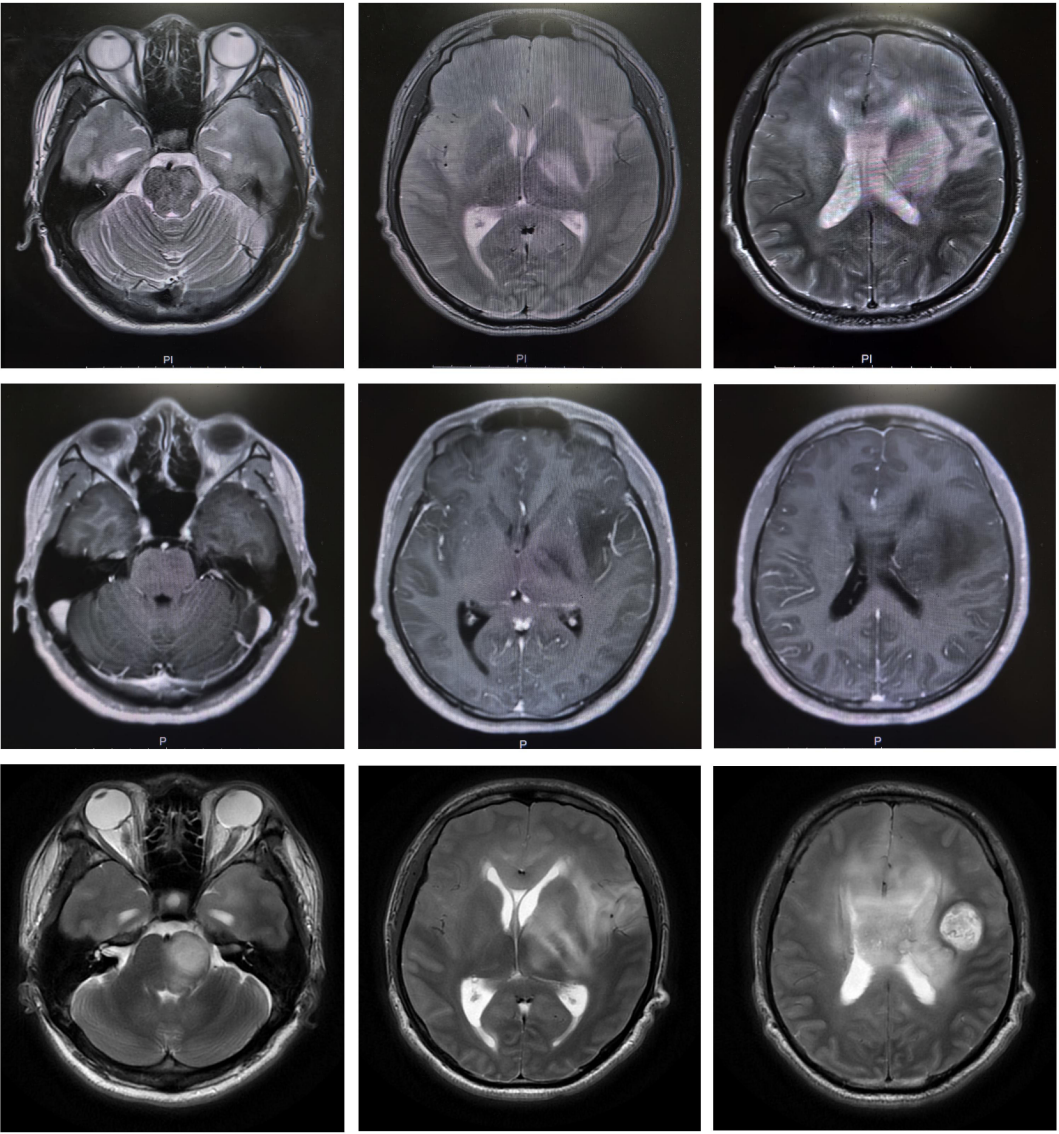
MRI manifestations of intracranial lesions in the patient: Panels (A1–3) show axial T2-weighted MRI images obtained at different levels during the patient’s initial onset in March 2024. Patchy hyperintense T2 lesions are observed in the bilateral frontoparietal lobes, the body of the corpus callosum, the left basal ganglia, thalamus, insular-temporal region, and pons. Panels (B1–3) present contrast-enhanced sequences; in image B3, linear enhancement can be seen within the lesion adjacent to the left lateral ventricle. Panels (C1–3) depict T2-weighted MRI images from a follow-up examination performed at our hospital in August 2024, showing enlargement of the lesion area compared with the prior scan, with a marked mass effect and newly developed nodular long T2 lesions along the inferior margin of the left frontal lobe and the body of the corpus callosum.
